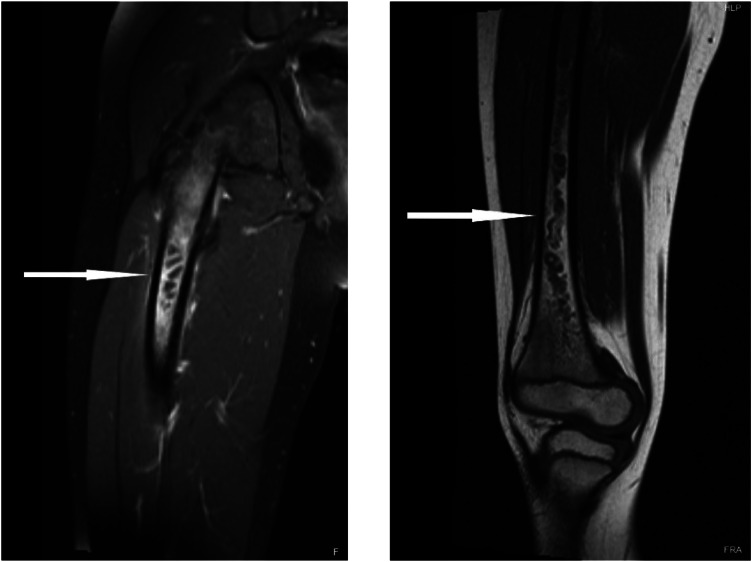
Magnetic resonance imaging of the right femur with contrast demonstrating lesions in the proximal (left) and mid (right) femoral diaphysis (arrows).
